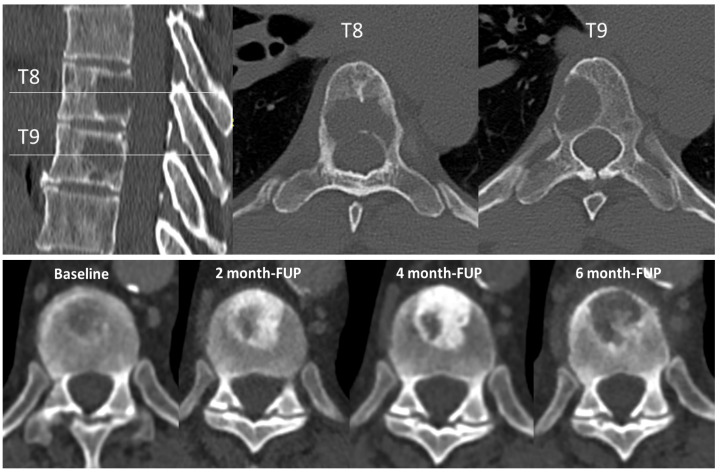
CT scan. Upper part: Multiplanar sagittal and axial reconstructions of the dorsal spine showing lytic lesions of myeloma involving T8 and T9 vertebrae. Lower part from left to right: progression of the size and condensation of a urothelial cancer bone metastasis under immunotherapy (avelumab), with initial lytic aspect and partial sclerosis of the lesion at 2 months. At 4 months, the sclerosis increases except in a focal region suspected of local progression. Confirmation of local progression with lysis of the sclerotic matrix at 6 months. FUP, follow-up.
